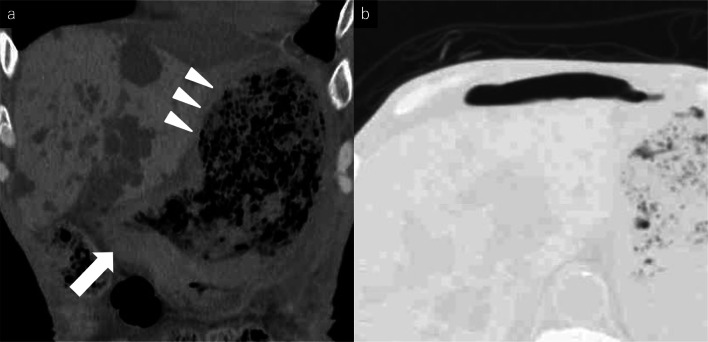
a Abdominal CT showed pyloric stenosis (arrow) and extensive gastric wall defects on the lesser curvature of the stomach (arrowhead). b A large amount of free air was observed around the liver
